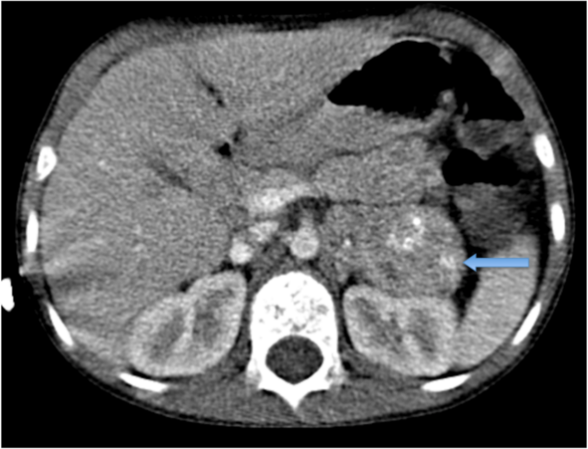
Axial CT of a 2 year old girl showing a left-sided abdominal NBL with evidence of calcification (blue arrows).
